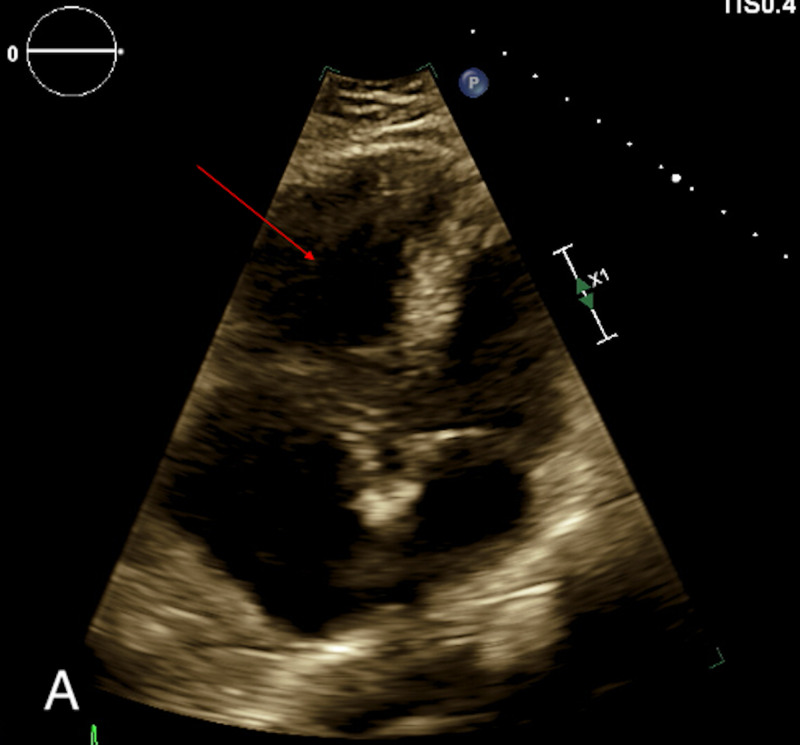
Initial transthoracic echocardiography of the patient described above. The right ventricle is severely dilated showing McConnell’s sign (red arrow).
